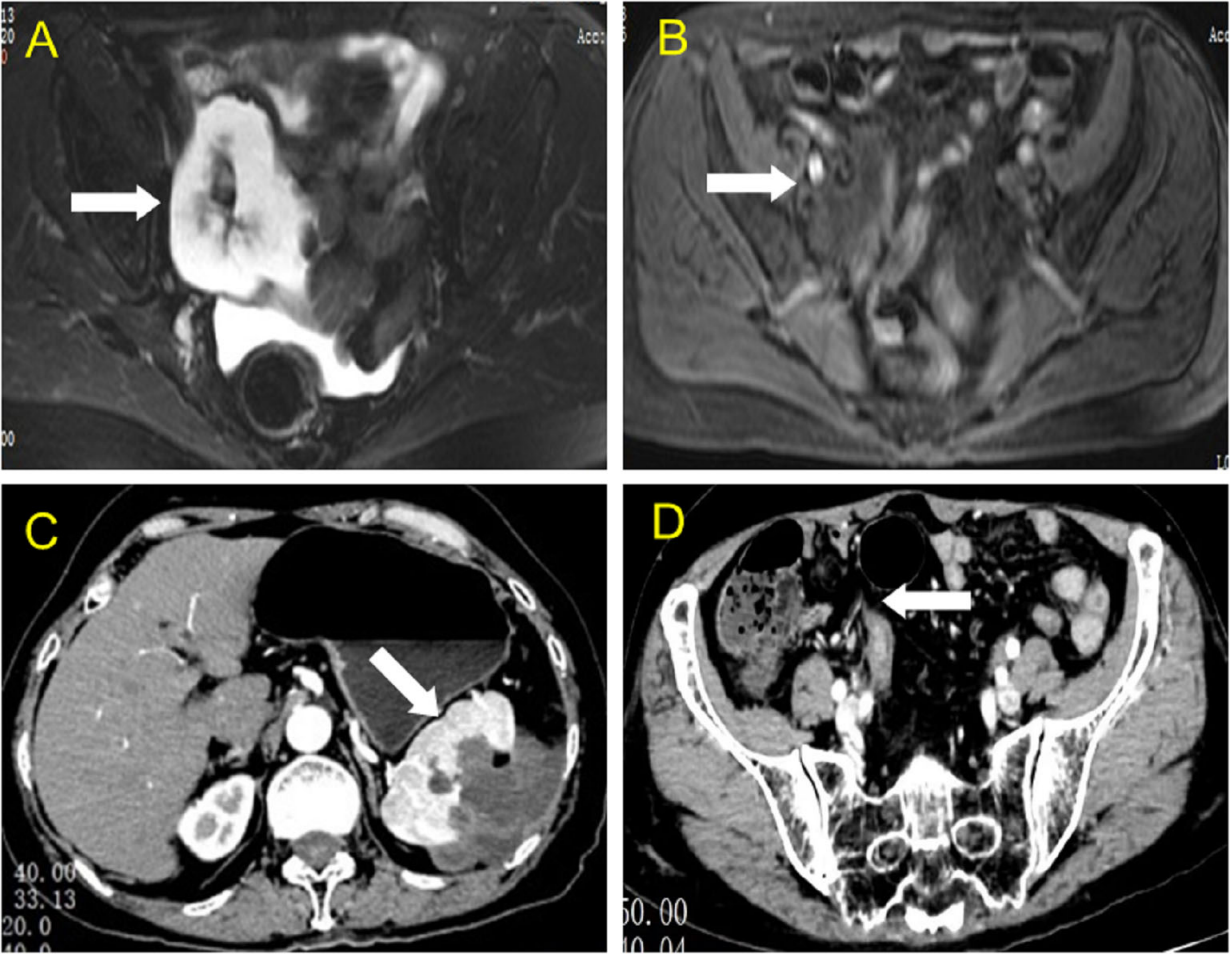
MRI and CT findings of the lesion in the right lower abdomen and spleen. (A, B) Axial view of the abdominal magnetic resonance scan revealed a slightly short T2 signal, a heterogeneous high signal in the diffusion-weighted image, and high signal intensity in the solid part (white arrow). (C) Axial view of contrast-enhanced computed tomography showing a multilocular cystic focus at the lateral splenic border (white arrow), which showed no significant enhancement after intracystic contrast perfusion, and intensity enhancement of the cyst wall after contrast perfusion. (D) Axial view of contrast-enhanced computed tomography revealed a mixed mass in the right lower abdomen, with significant enhancement in the central solid part.
